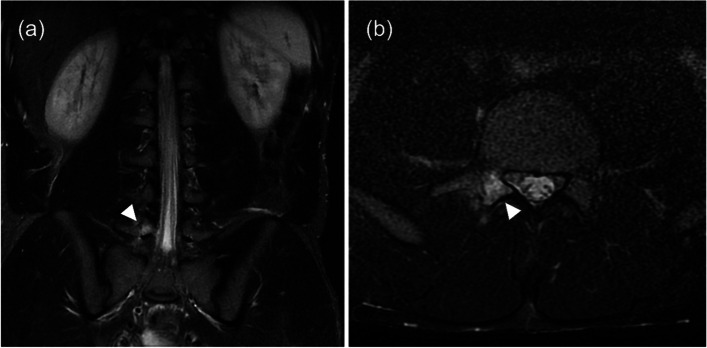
A football player diagnosed with a stress reaction of the right L5 pars interarticularis. The increased T2 signal intensity was detected on (a) coronal and (b) axial STIR images of MRI (arrows)
